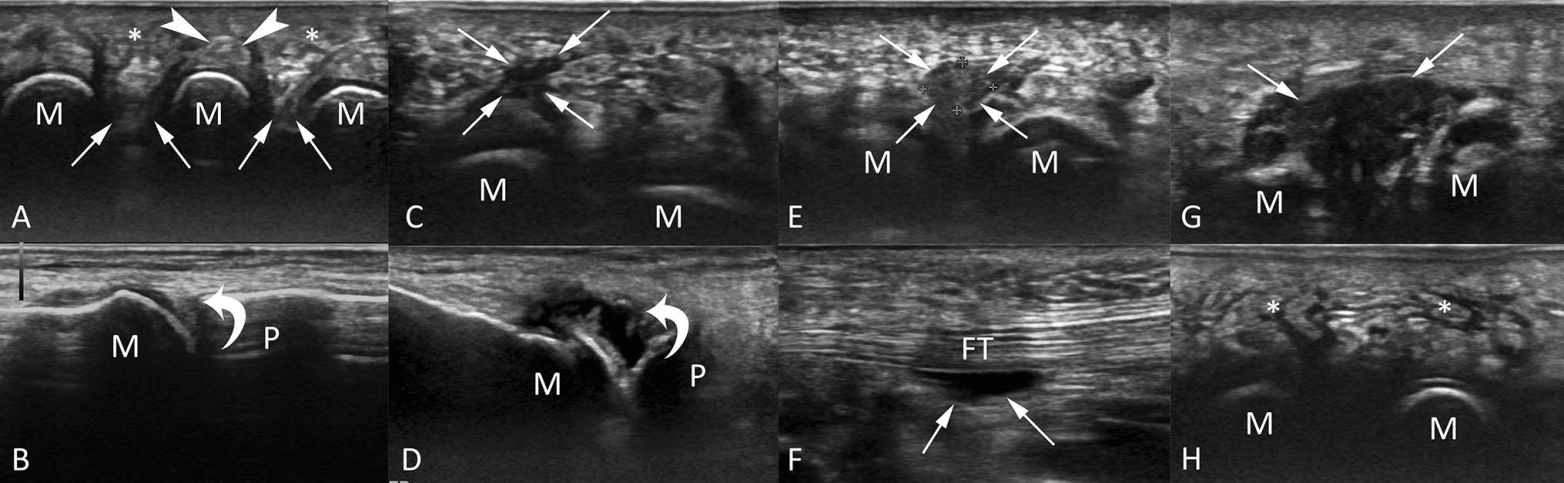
Ultrasound findings of some representative cases from our study population. Transverse image with plantar approach a of a patient with normal anterior plantar fat pad (asterisks), flexor tendon (headarrows), and intermetatarsal spaces (arrows). Longitudinal image with dorsal approach b of the first metatarsophalangeal joint with no effusion (curved arrow). Transverse image with plantar approach of submetatarsal bursitis (c, arrows). Longitudinal image with dorsal approach d of the first metatarsophalangeal joint with pathological effusion (curved arrow). Transverse image with plantar approach of Morton’s neuroma (e, arrows). Longitudinal image with plantar approach of flexor tendinitis (f, arrows). Transverse image with plantar approach of intermetatarsal bursitis (g, arrows). Transverse image with plantar approach h of a patient with oedema of the anterior plantar fat pad (asterisks). M = metatarsal; P = proximal phalange; FT = flexor tendon
